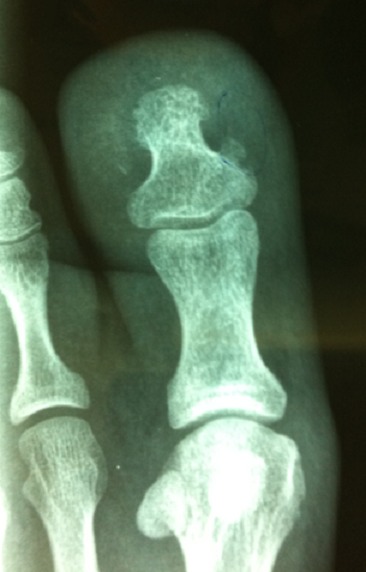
Radiographie de l’hallux de face montre une base d’implantation plus ou moins large de même tonalité osseuse que la phalangette
